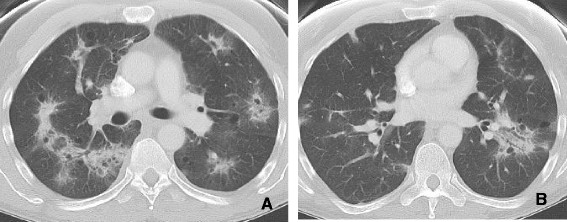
COP. A and B: CT scan shows associated poorly defined nodular areas of consolidation and cavitation along the bronchovascular bundle. Presence of centrilobular nodules and associated bronchial dilatation. Findings are consistent with COP (Cryptogenic organizing pneumonia).
